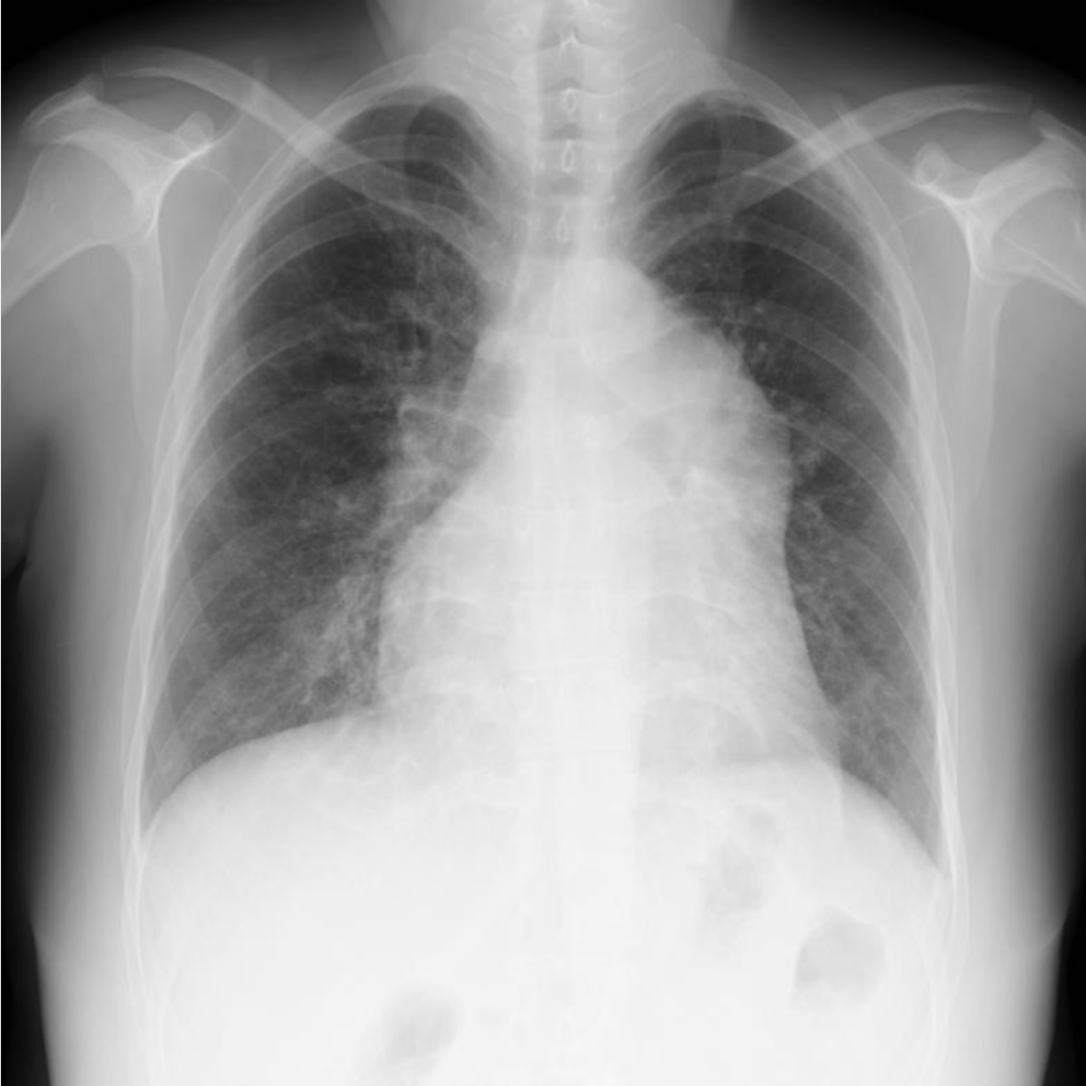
Preoperative chest radiograph. The image shows an enlarged mediastinal shadow
